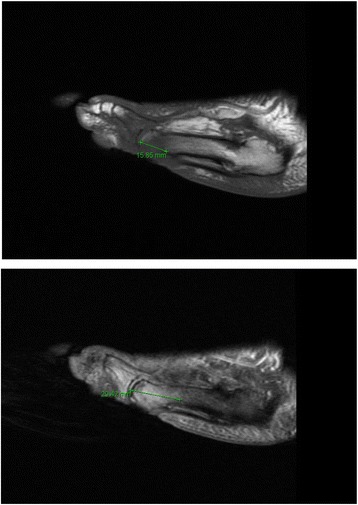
The top photograph displays a sagittal view of a T1 MRI image. The green line represents the distance of the proximal OM propagation. The bottom photograph displays a sagittal view of a T2 MRI image. The green line represents the distance of the proximal bone marrow edema propagation
